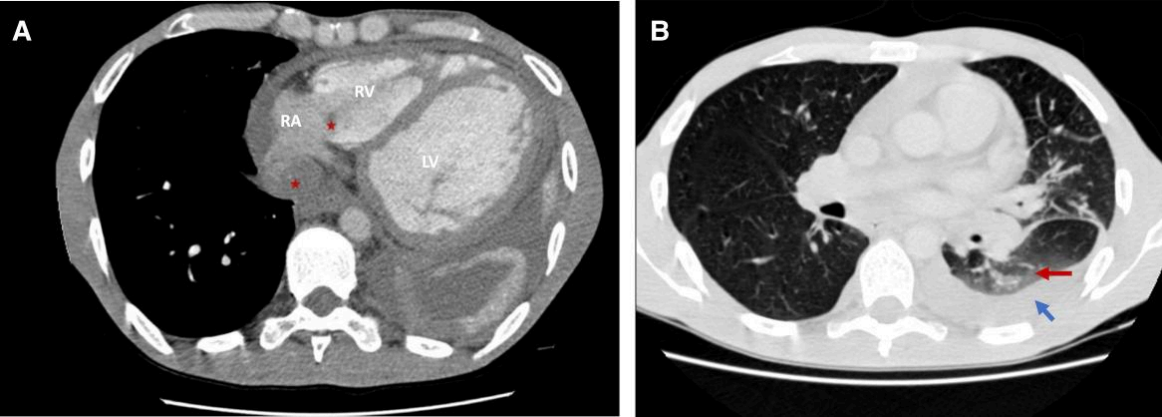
(*A*) Axial thoracic scan showing the same thrombi seen on transthoracic echocardiography (red stars) as well as cardiomegaly and dilatation of the left ventricle. (*B*) Axial thoracic scan showing ground glass (red arrow) opacities and pleural effusion (blue arrow). RA, right atrium; RV, right ventricle; LV, left ventricle.
